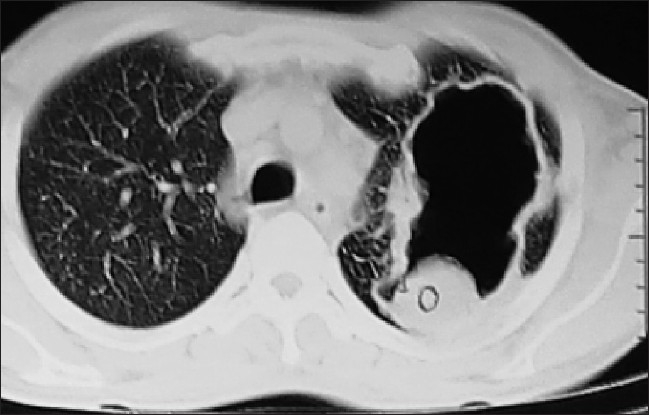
CT scan of the chest showing a crescent shaped lucency (air crescent sign) with in the area of consolidation left upper lobe
